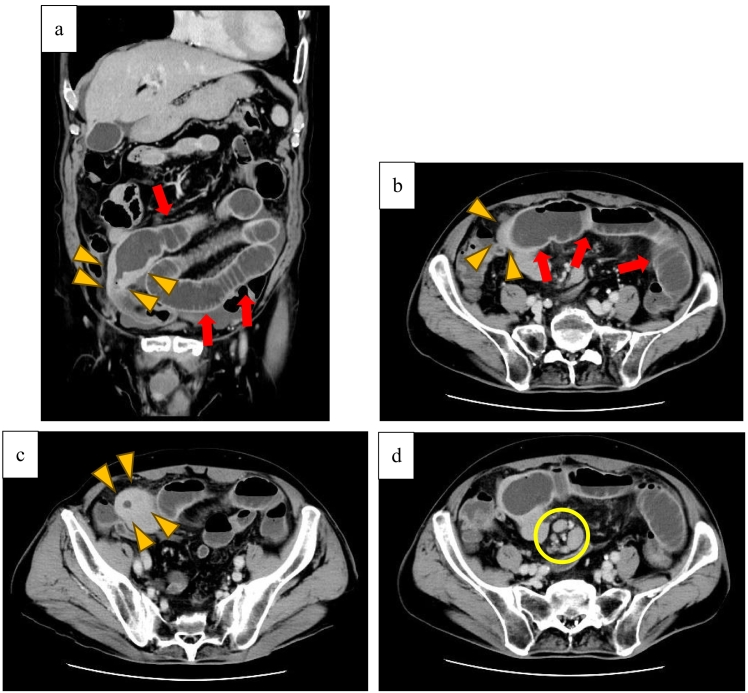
Contrast-enhanced CT revealed wall thickening of the small intestine (arrowhead) and marked bowel dilation and fluid retention on the oral side of the wall thickening (arrow). Enlarged mesenteric lymph nodes were also observed (circle). (a) Coronal CT image. (b)–(d) Axial CT images.
